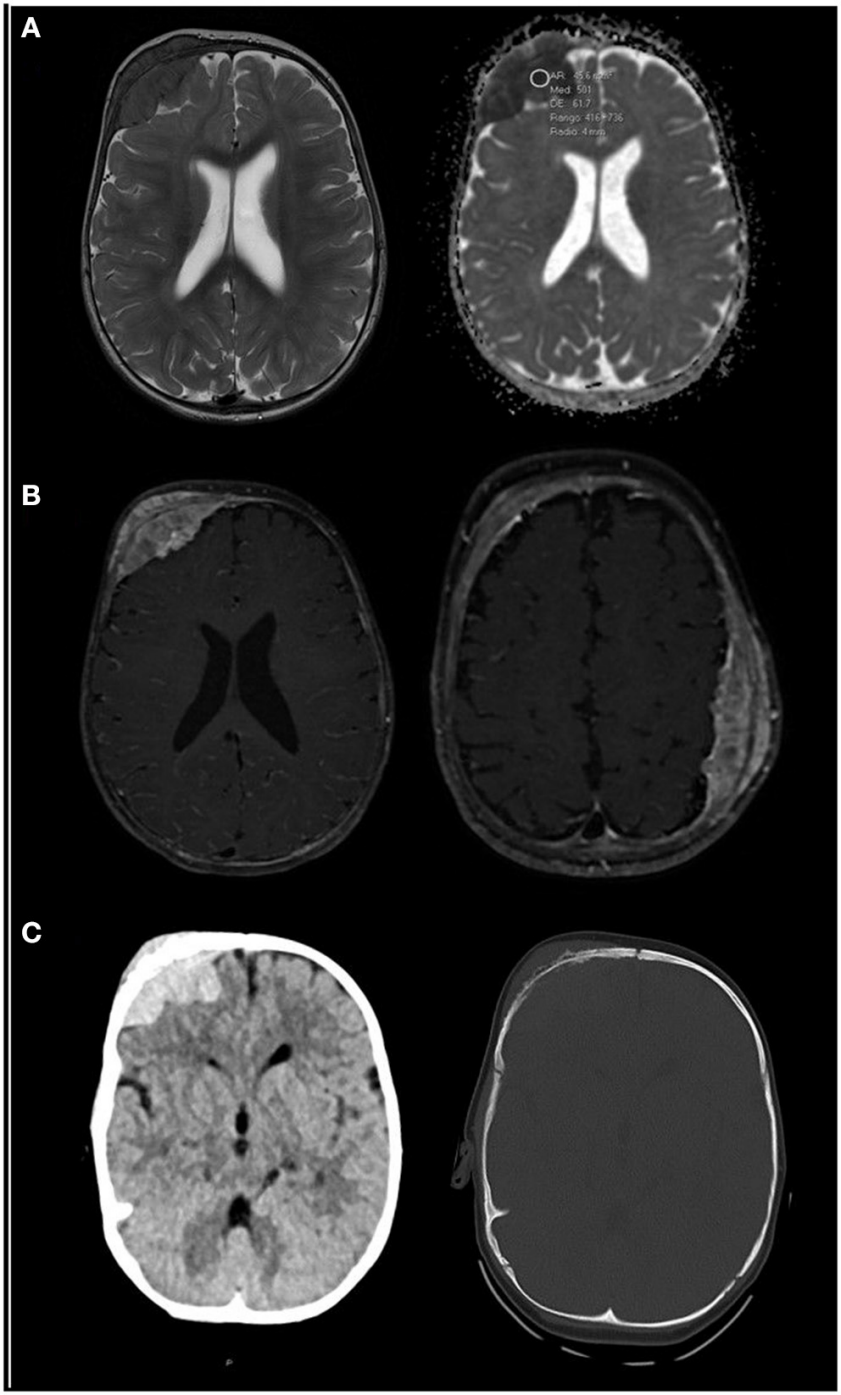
(A) Axial T2-weighted (right image) and apparent diffusion coefficient (ADC, left image) MRI sequences. A right frontal soft tissue mass is patent, hypo-intense on T2 and with low ADC values (500), which likely represent high cellularity. (B) Axial contrast-enhanced T1-weighted images with fat saturation at two levels show bilateral masses, right frontal and left parietal, with homogeneous enhancement. (C) Unenhanced CT scan centered in the right frontal mass shows spontaneous hyper-density in the parenchymal window (right image), also typical of hyper-cellular tumors. Bone window (left image) shows erosive changes within the subjacent bone. Findings are consistent with myeloid sarcoma. MRI scans were taken in December 2020.
